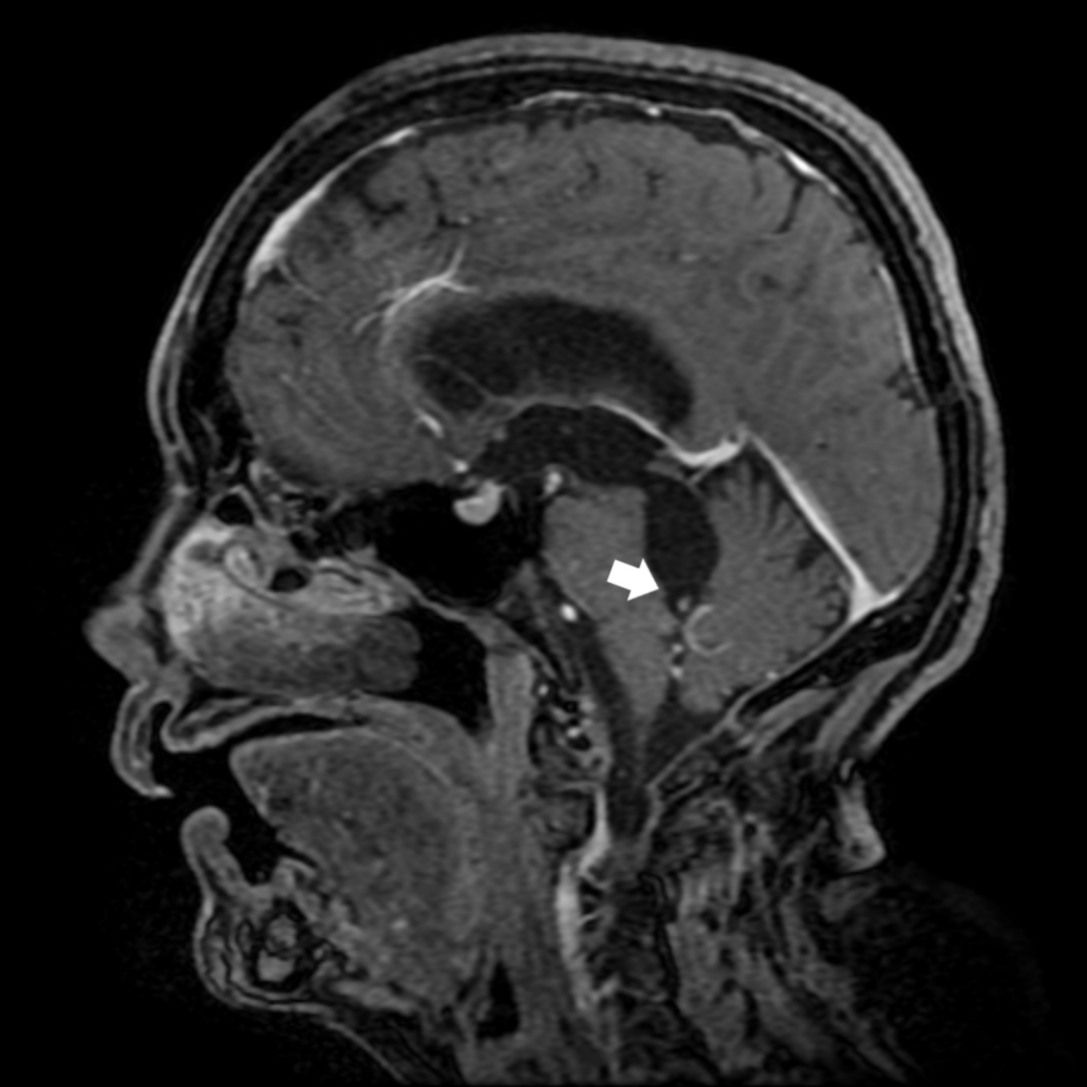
MRI T1-weighted sagittal. T1-weighted sagittal MRI image showing the presence of cysticerci causing obstruction of the Magendie's foramen.
